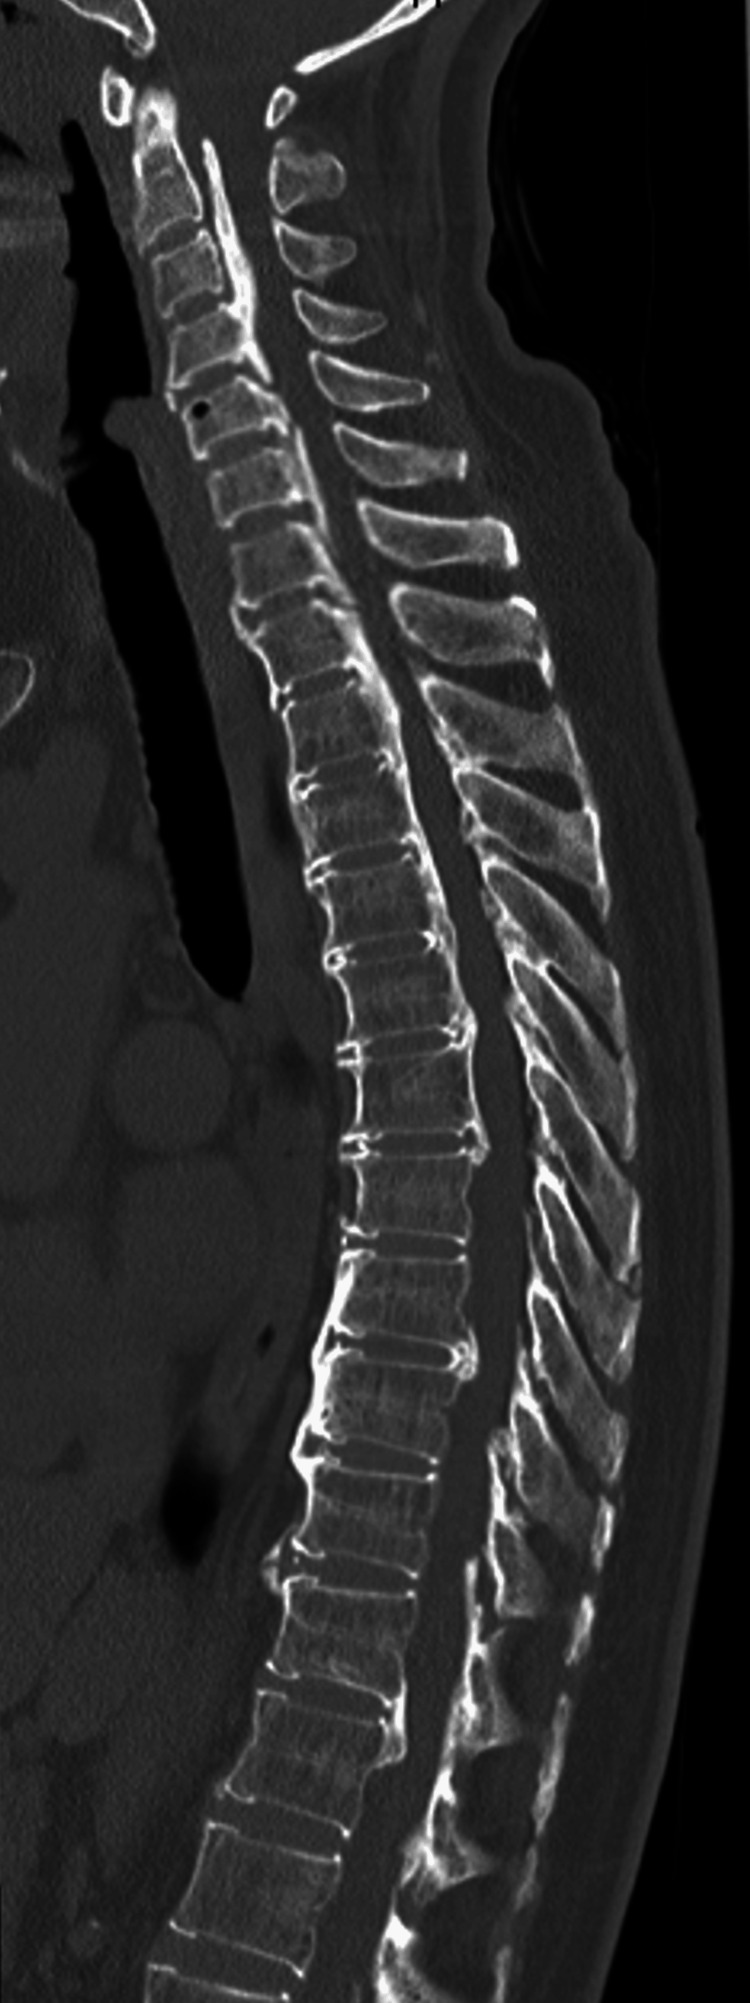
Computed tomography showed ossification of the posterior longitudinal ligament at C2-Th6, Th8/9, and Th11/12.
